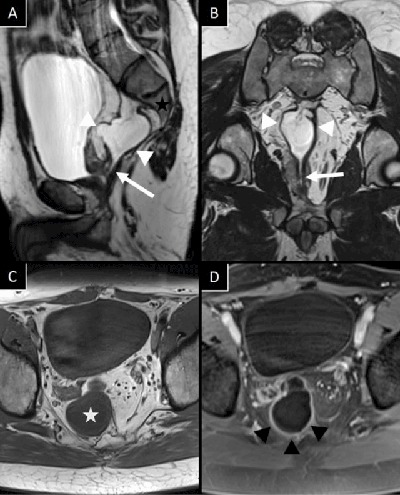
Sagittal (A) and paracoronal reformatted (B) T2-weighted images show a large thick-walled presacral cystic mass (arrowheads) with a thin anteroinferior neck (arrows) connecting to the prostatic urethra at the level of the verumontanum. The cystic component has a homogeneous high T2 signal intensity, with a low T2 signal intensity cyst wall. Axial T1-weighted image (C) shows low signal intensity of the cyst (white asterisk), similar to the bladder content, excluding hemorrhagic/proteinaceous components. Axial fat saturated T1-weighted vibe image after intravenous contrast administration (D) shows only contrast enhancement of the cyst walls (black arrowheads). There is a right-sided displacement of the prostate secondary to surgical pull-through intervention as treatment for the anal atresia. Also note the congenital sacral deformity (black asterisk on A).
